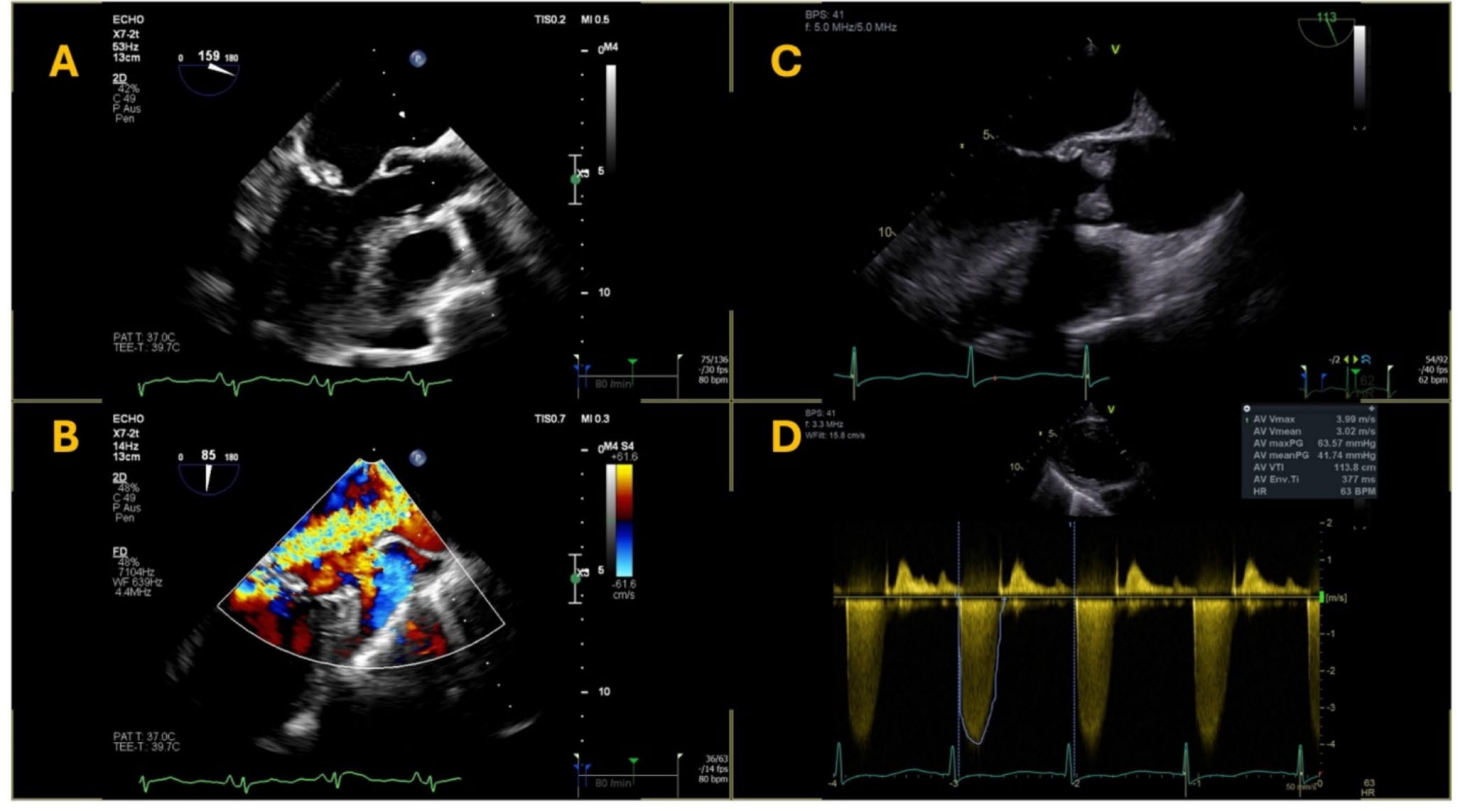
Transesophageal echocardiography findings of two PVIE patients from the analyzed cohort. One patient with native mitral valve endocarditis with a large vegetation on the posterior mitral valve leaflet (Panel A) and severe mitral regurgitation. (B) The other patient with prosthetic aortic valve endoplastitis (C) and severe valvular stenosis caused by large vegetations (D)
